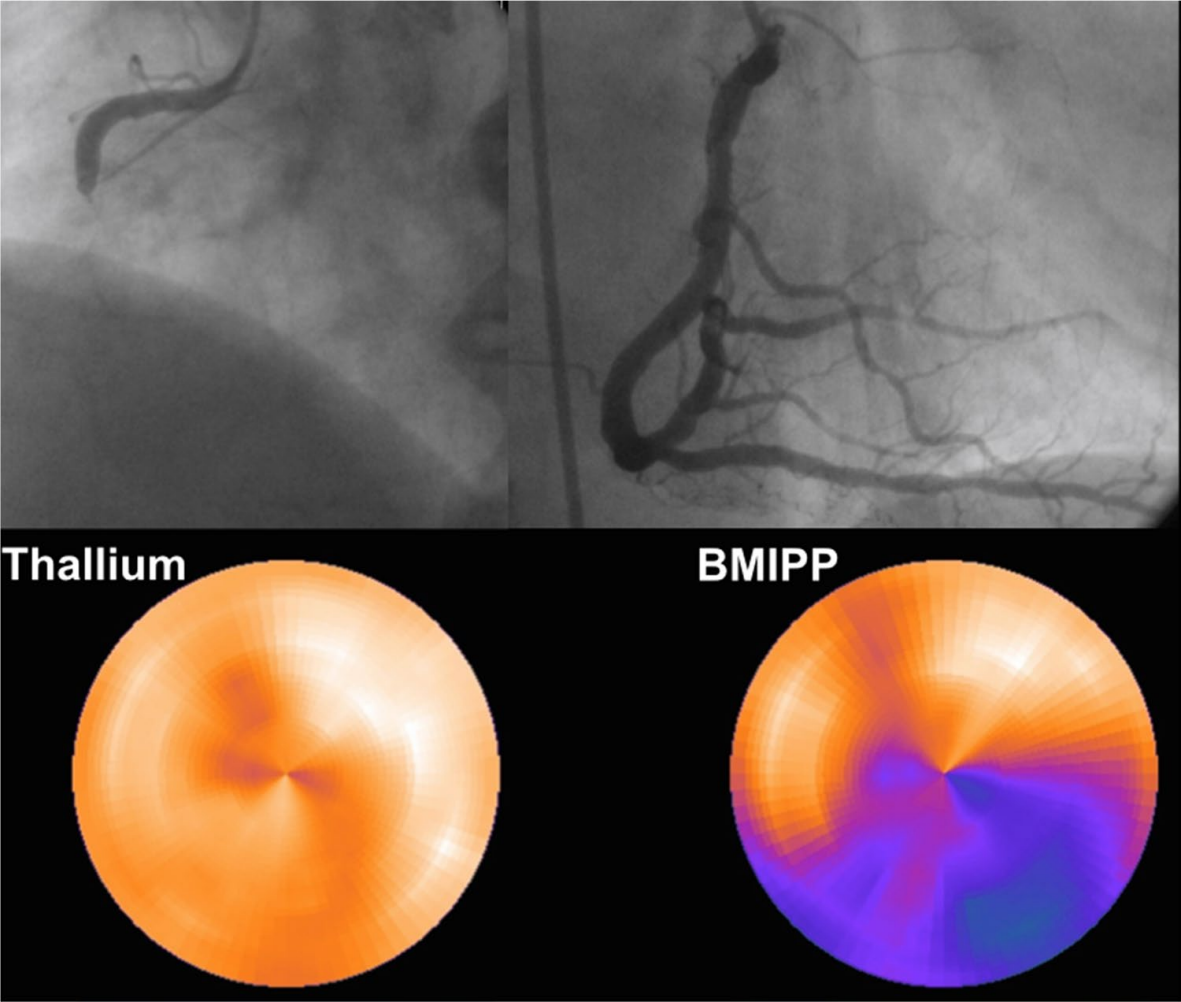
Coronary angiography (CAG) before and after the completion of primary coronary intervention (PCI) and dual-loading scintigraphy with thallium-201 and iodine-123 BMIPP before discharge. Upper left panel: CAG prior to PCI. The proximal right coronary artery (RCA) is completely occluded. Upper right panel: CAG after completion of PCI. Lower panel: Bull’s eye displays of dual-loading scintigraphy with thallium-201 and iodine-123 BMIPP before discharge. The take-up of thallium by the myocardium supplied by the RCA was excellent, in stark contrast to the large defect observed in BMIPP imaging. Reprinted from Koyama et al. [57]
